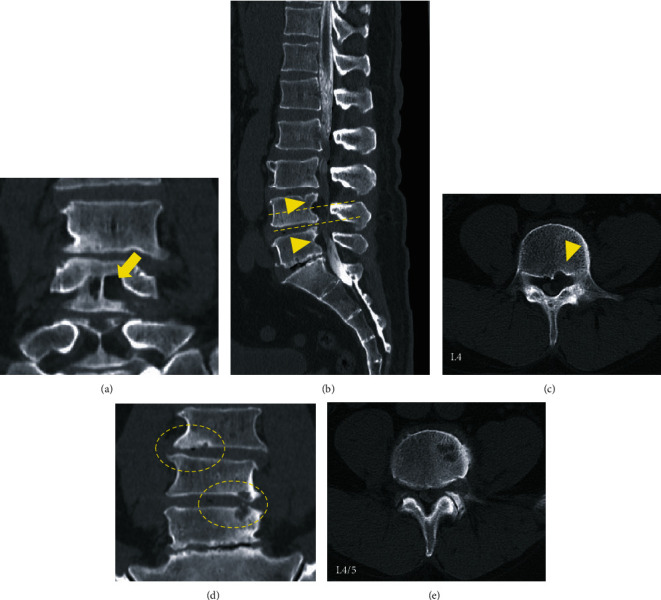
Computed tomography scans in coronal (a, d), sagittal (b), and axial (c, e) views showing posterior vertebral scalloping changes of L3, L4, and L5 without erosion of the cortical and medullary bone (arrowheads) and the cavity of the posterior wall connecting the foramen (arrow). Schmorl's nodes at the endplates of L3, L4, and L5 are observed (circle).
